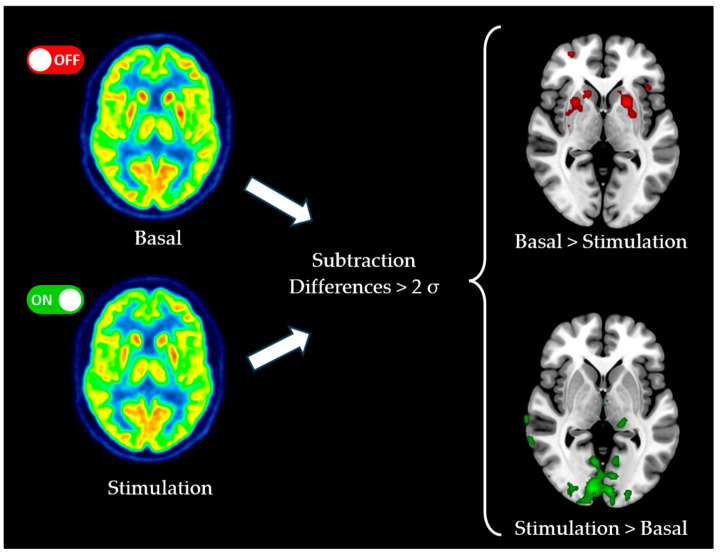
Summary of the PET subtraction algorithm applied in our study. Subtraction maps are presented over a standard MRI image.
